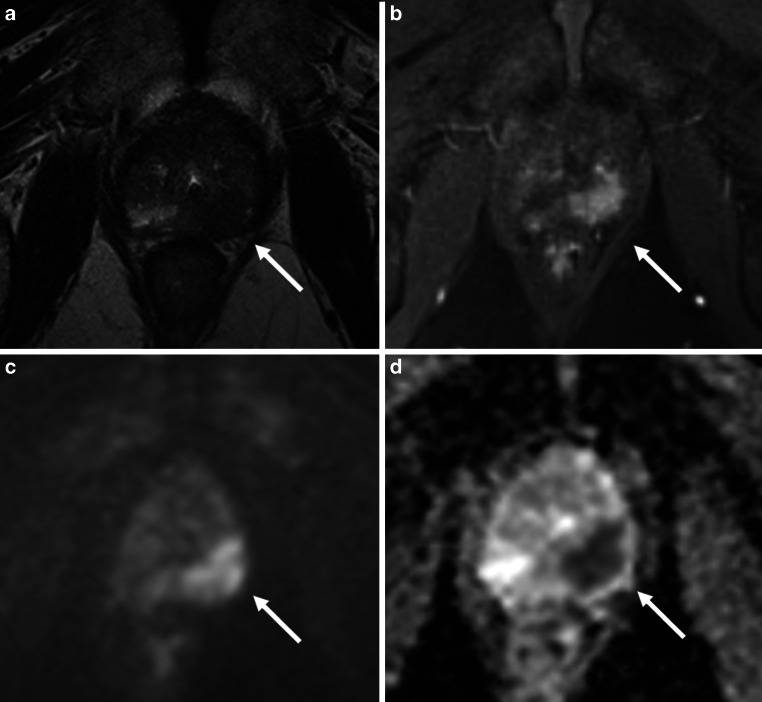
Prostate cancer multiparametric magnetic resonance imaging (mpMRI): mpMRI of a prostate cancer located in the peripheral zone left (indicated by arrow, axial diameter 19 mm), Prostate-Imaging - Reporting and Data System (PI-RADS) score 5. Capsule contact 30 mm, capsule bulging, and irregular capsule margins, resulting in an mpMRI-based extra-prostatic extension (mEPE) score of 5. (mpMRI sequences: a T2-weighted, b dynamic contrast-enhanced MR perfusion, c diffusion-weighted magnetic resonance imaging, d apparent diffusion coefficient)
